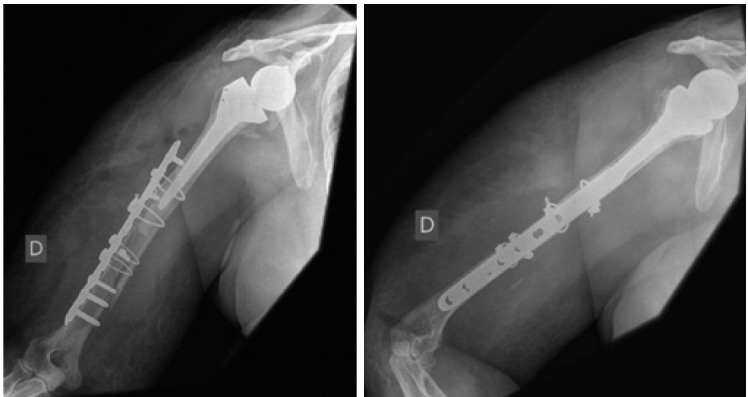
Front and profile radiography showing osteosynthesis with plates, screws and cables immediate after surgery
